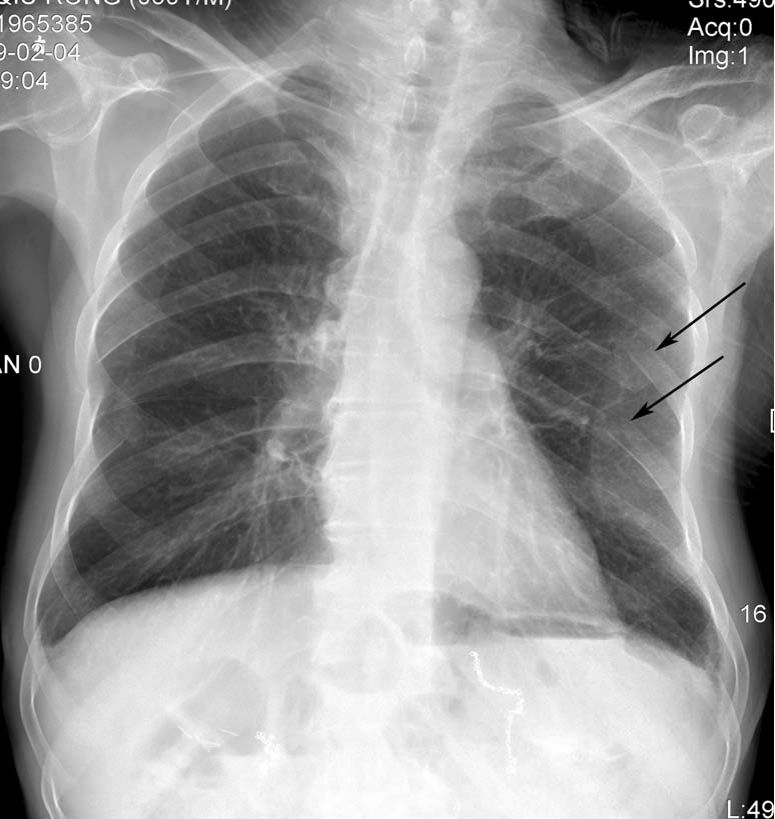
X-ray image. A chest radiograph showed fractures of the left sixth and seventh ribs (arrow).
